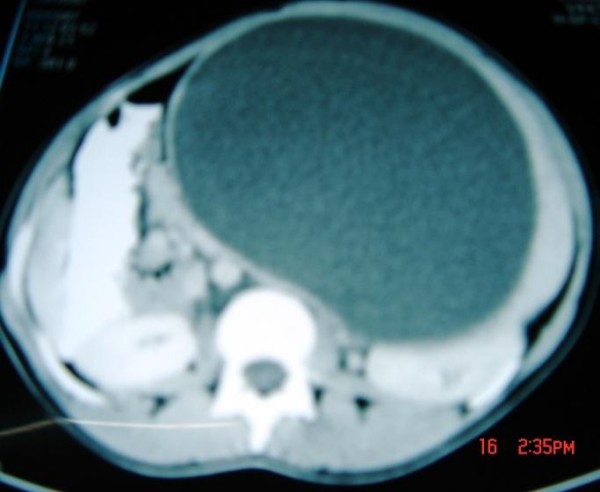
Abdominal CT scan showing a giant splenic pseudocyst with net lining, and a diameter of 20 cm.
